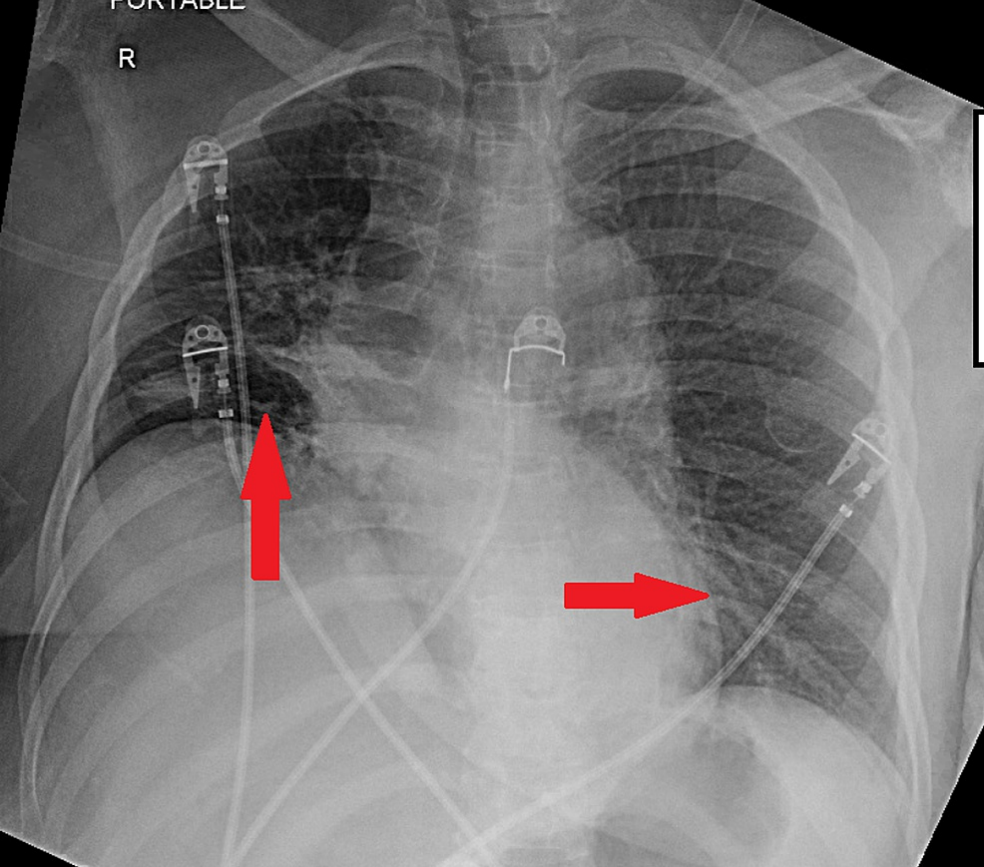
Portable chest radiograph indicating right perihilar, right lower lobe, and left lower lung airspace opacities (red arrows), consistent with multi-segment or viral pneumonia.
